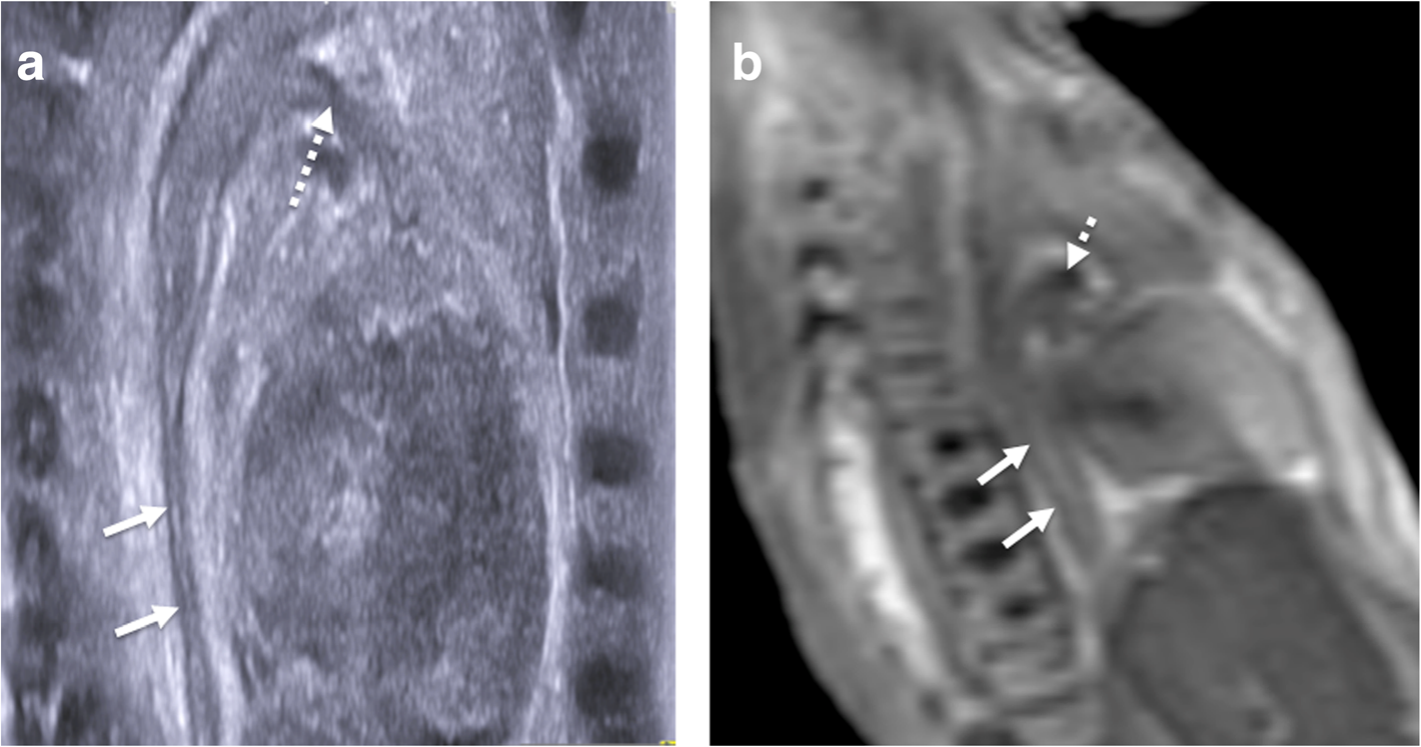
Paired sagittal midline imaging of the chest, in a 20-week gestational-aged foetus obtained approximately 1 week after death. The postmortem ultrasound imaging (a) and T2-weighted MRI imaging at 1.5 T (b) both clearly demonstrate the usual appearances of haemostasis and collapse of the descending aorta (solid white arrows) and also prominent patent ductus arteriosus (dotted arrow)
